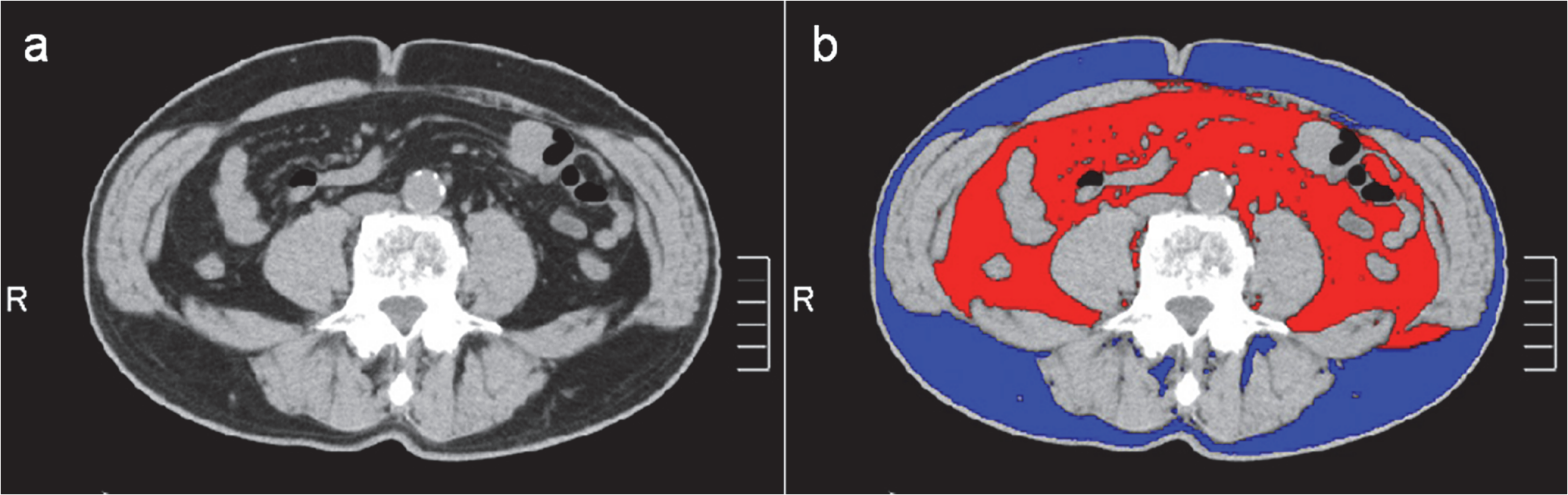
Measurement of intra-abdominal fat area by use of multidetector CT. A 61-year-old-male with a BMI of 24.8. (a) Initial CT images. (b) Intra-abdominal adipose tissue areas. Regions of red and blue color indicate visceral (107.3 cm^2^) and subcutaneous (104.1 cm^2^) adipose tissue, respectively.
